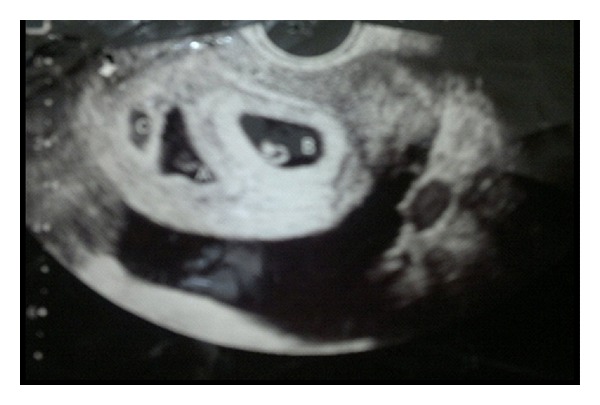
Ultra Sonography showing three gestational sacs with gross ascites.
